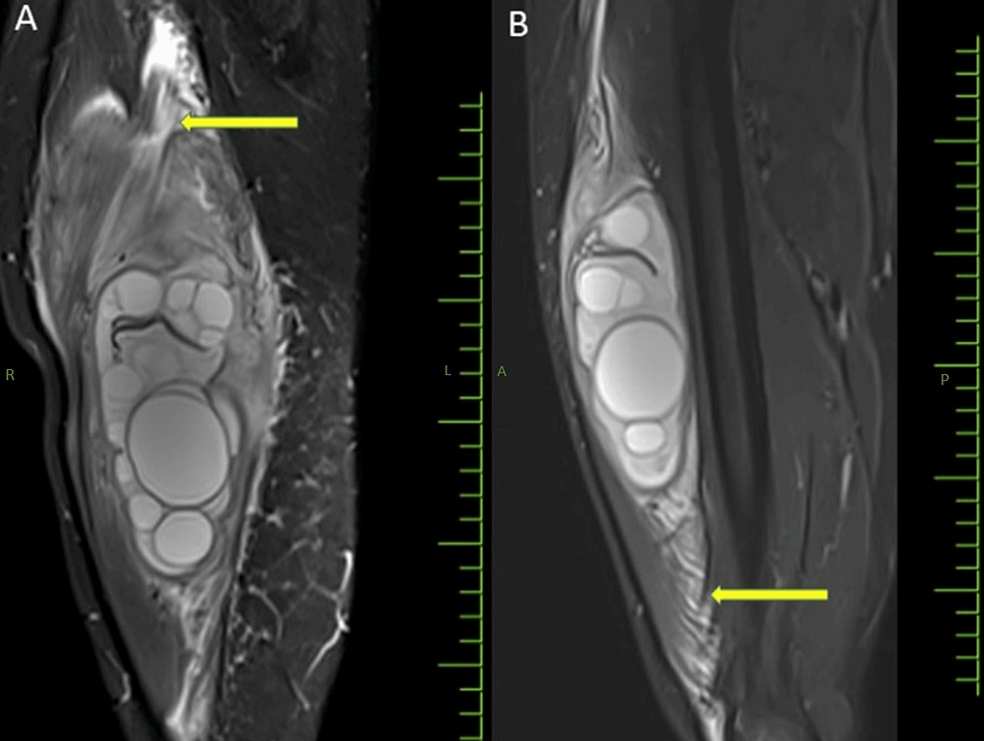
T2-weighted STIR MRI of the thigh: (A) coronal and (B) sagittal images showing ill-defined hyperintensities in the surrounding muscles adjacent to the hydatid cyst suggestive of inflammatory changes (yellow arrows). MRI, magnetic resonance imaging; STIR, Short Tau Inversion Recovery
